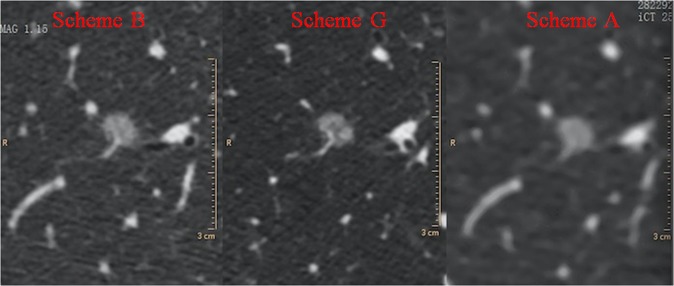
Local enlarged nodule images for the three CT scanning schemes. Scan scheme G improves spatial resolution and provides better detail detections of nodule structure.
